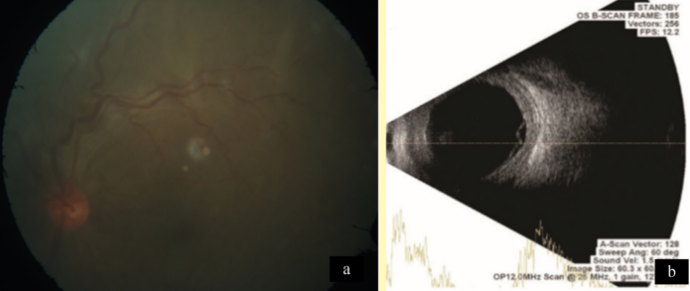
a) Fundus of the left eye showing exudative retinal detachment. b) B-scan ultrasonography showing exudative retinal detachment with thickened choroid and fluid in sub-Tenon’s space.
